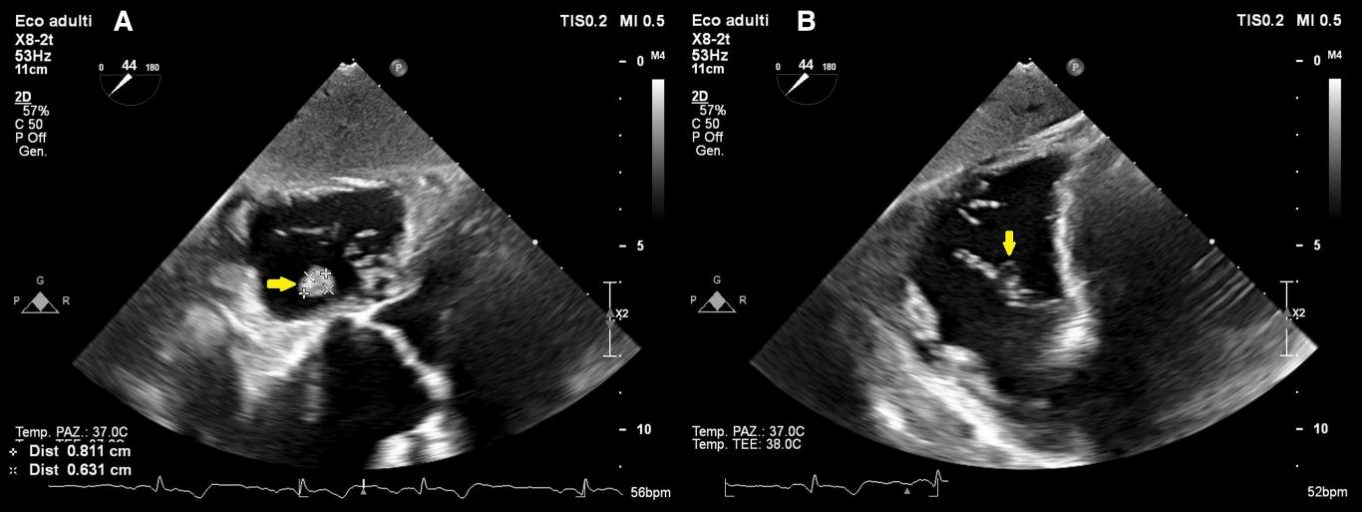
(*A*) Short axis view of the tricuspid valve from the transoesophageal transgastric window, showing an oval hyperechoic mass attached to the anterior leaflet. (*B*) Off-axis view of the same window revealing an internally empty mass.
